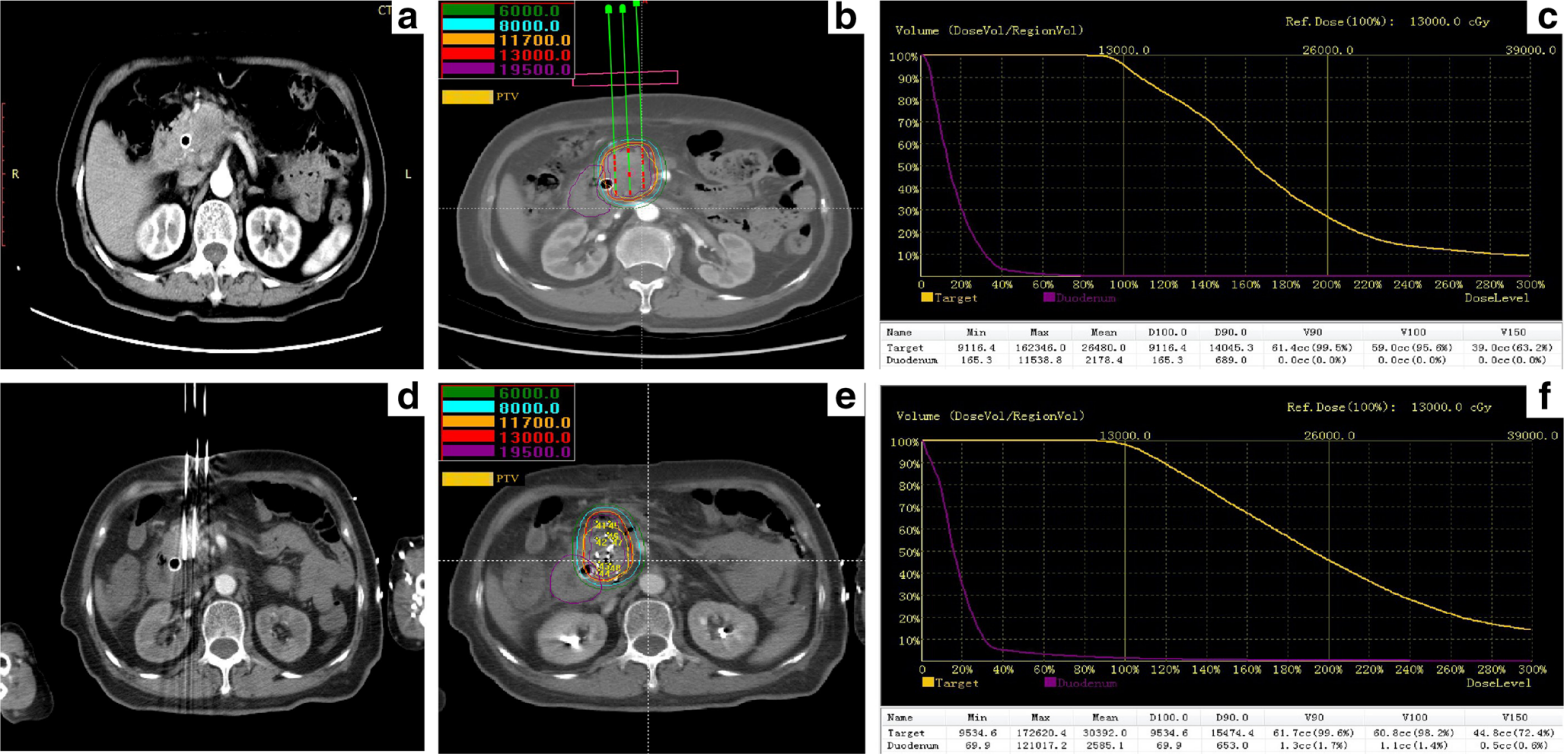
CT-guided percutaneous iodine-125 seed brachytherapy. a Preoperative computed tomography (CT) scan. b, c Treatment plan and the dose-volume histogram calculated before brachytherapy, the prescribed dose of this patient was 130 Gy. d Intraoperative puncture procedure under the CT-guided. e, f Coverage and the distribution of implanted seeds, dose distribution on treatment planning system and the dose-volume histogram calculated after brachytherapy. D90 was 154.74Gy and V100 was 98.2%. PTV, planning treatment volume; D90, the dose covering 90% of the target tumor volume; V100, the percentage of the target tumor volume covered by 100% prescribed dose
